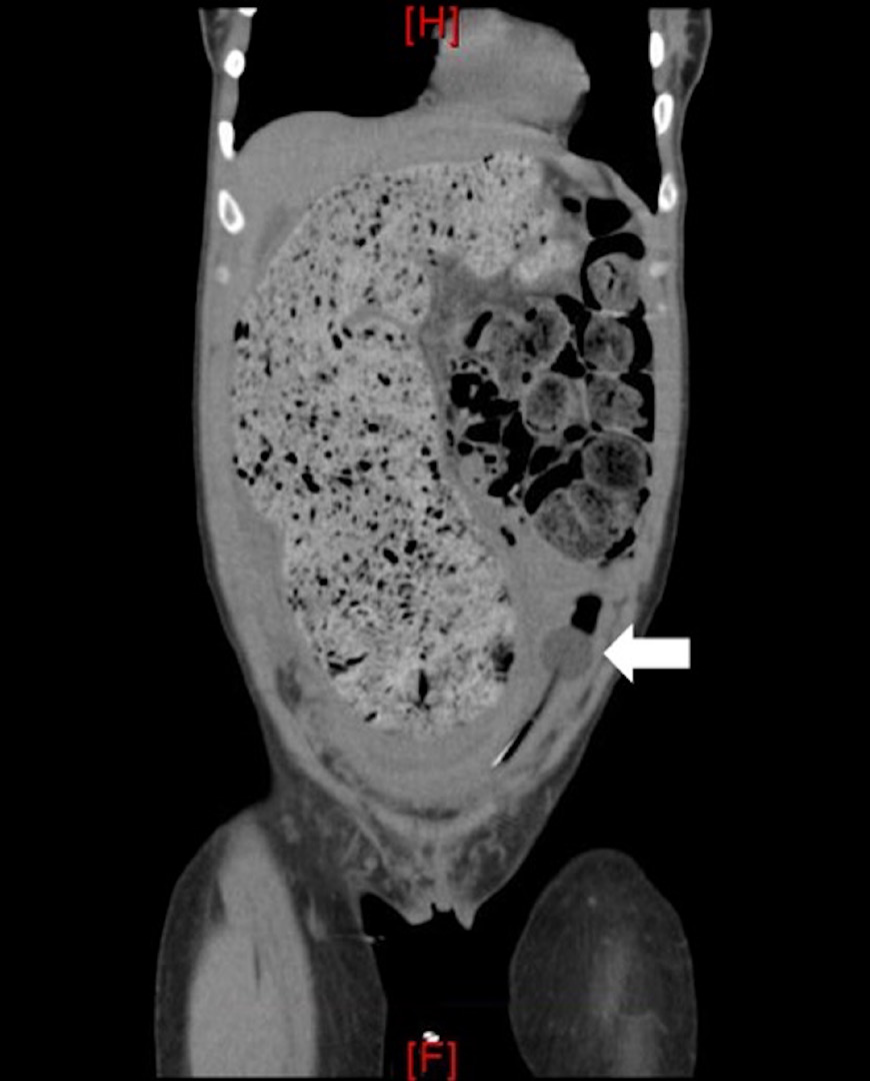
Computed tomography of the abdomen in the coronal plane demonstrating significant stool burden with displacement of the bladder to the left as noted by the urinary catheter bulb (arrow).
